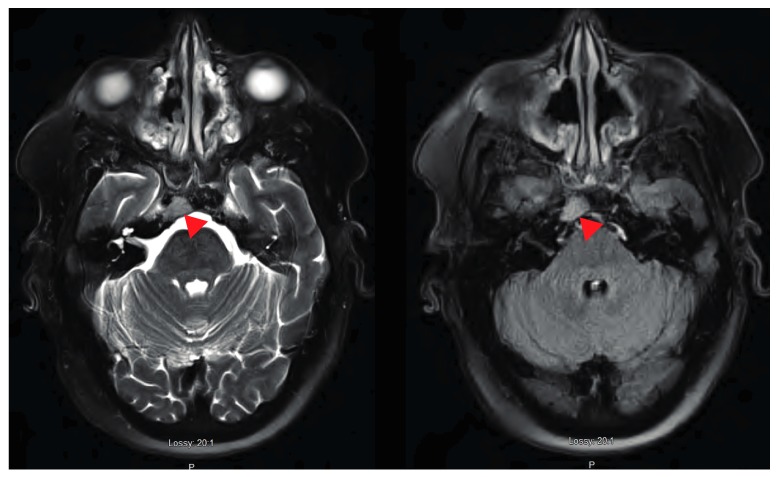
Axial T2 FS repeat (left) and T2 (right) demonstrating a discrete lesion (indicated by red triangle) in the same region as CT scan. Lesion is seen on the right clivus adjascent to Dorello’s canal and is likely responsible for CN 6 palsy. Lesion suspicious for myeloma and metastases. However, given the signal intensity and restricted diffusion lymphoma is also a possibility.
